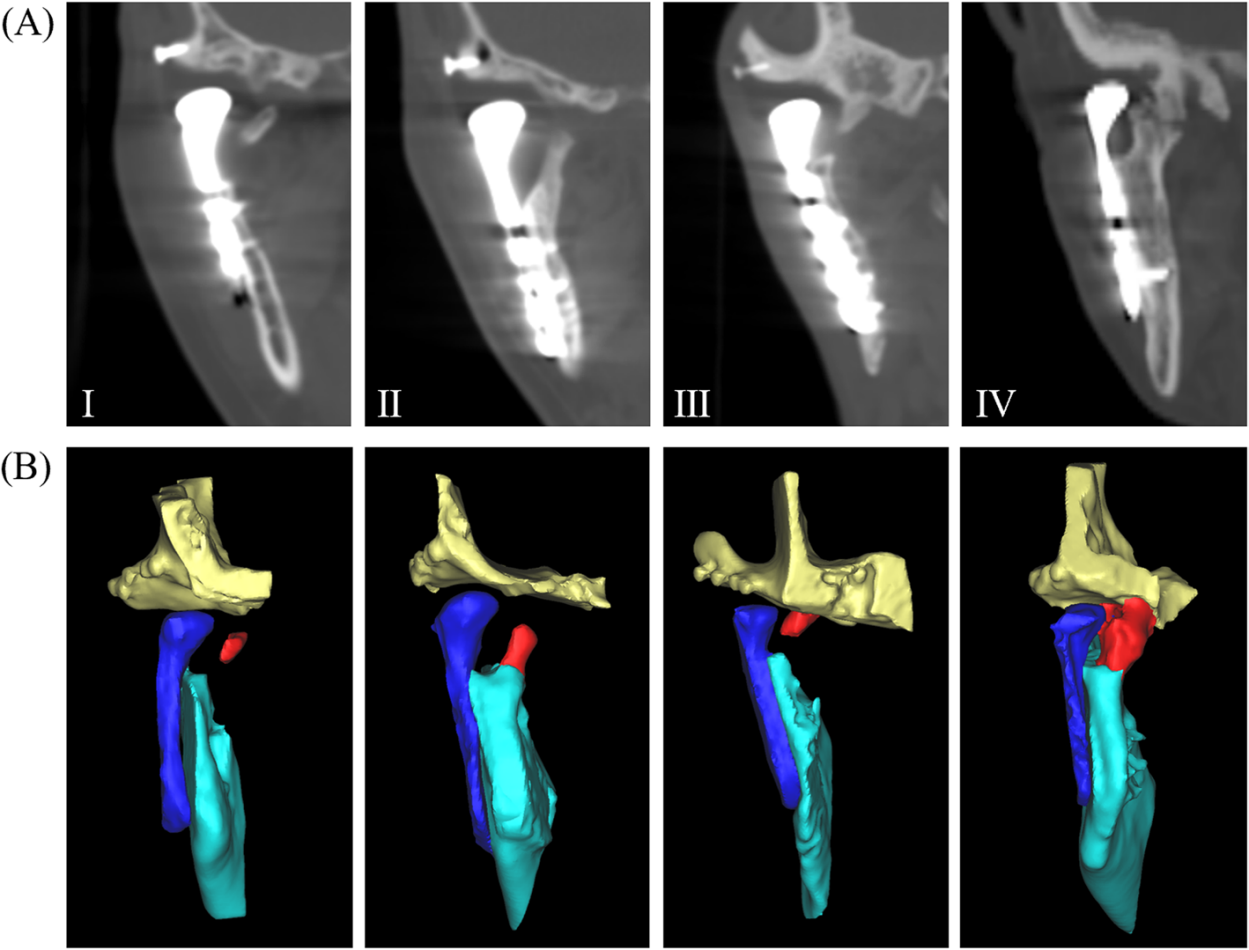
The classification of heterotopic ossification (HO) after alloplastic temporomandibular joint replacement. A coronal reconstruction of CT, B three-dimensional reconstruction of CT. Red, HO; dark blue, prosthesis; blue, mandible; yellow, skull base
